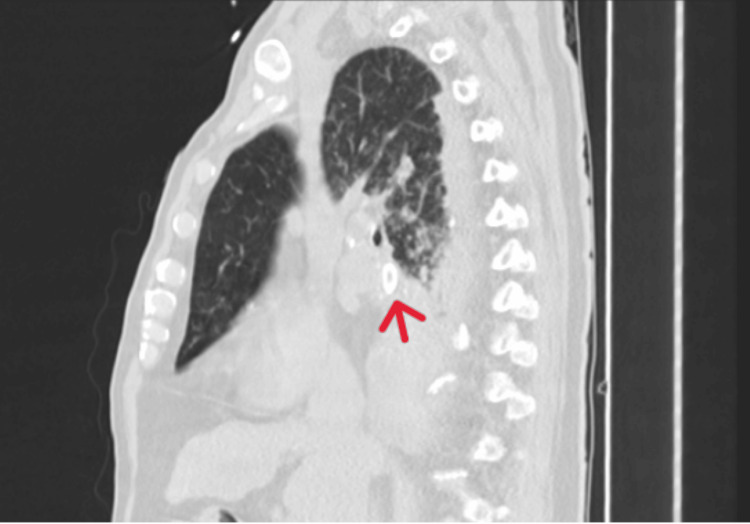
Coronal plan of the chest CT scan showing an oval structure with coarse peripheral calcification, with approximately 19 mm of longest axis (red arrow)
